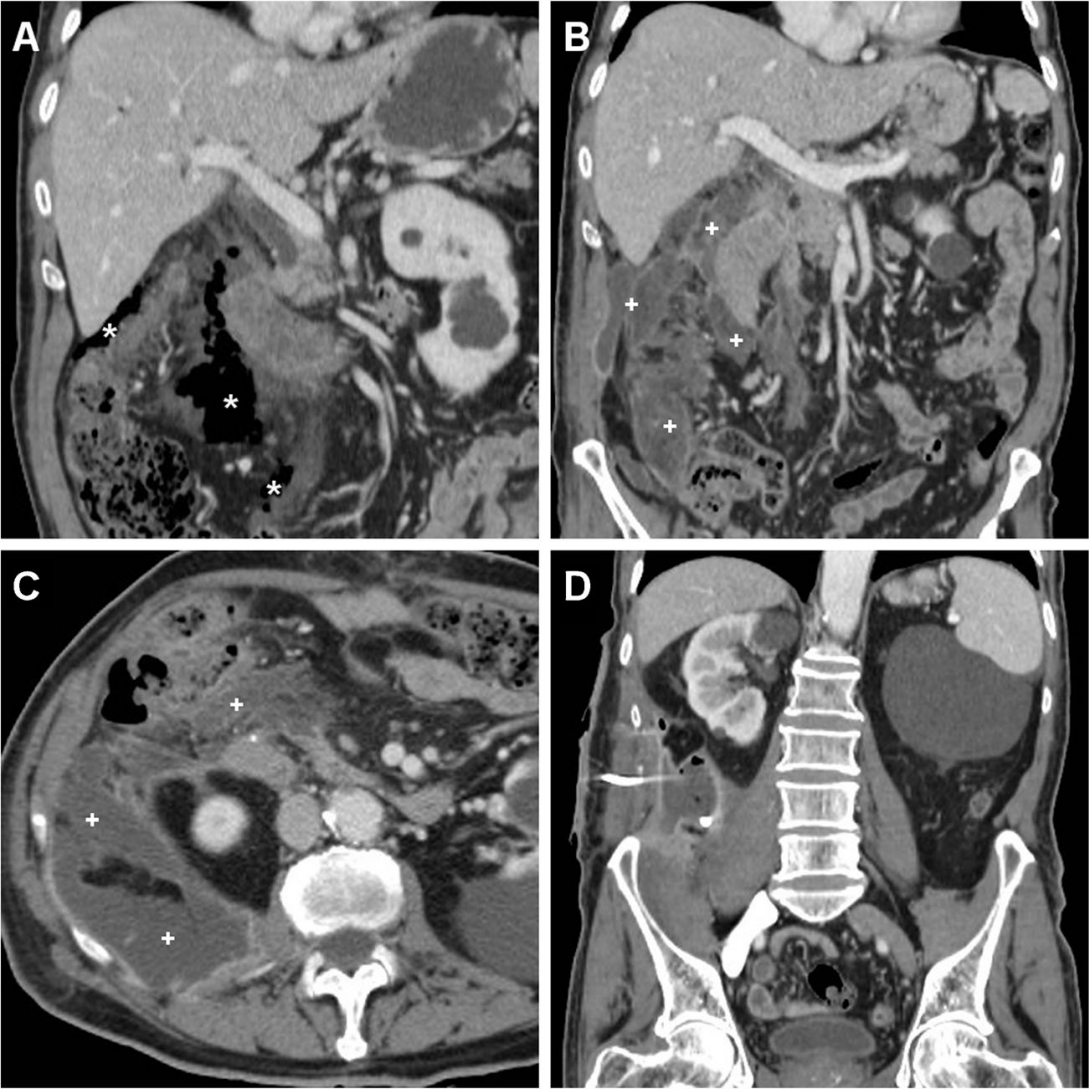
Failed conservative treatment of DP in an 83-year-old male with chronic duodenal stricture who underwent ERCP to prevent further episodes of infectious cholangitis. The next day, contrast-enhanced CT (a) showed moderate right-sided retroperitoneal gas (*). Follow-up CT (b, c) 4 days later showed formation of multiple confluent periduodenal and right parietocolic fluid-like infected collections (+), with extensive occupation of the ipsilateral posterior pararenal space, which required percutaneous drainage (d) to relieve sepsis
